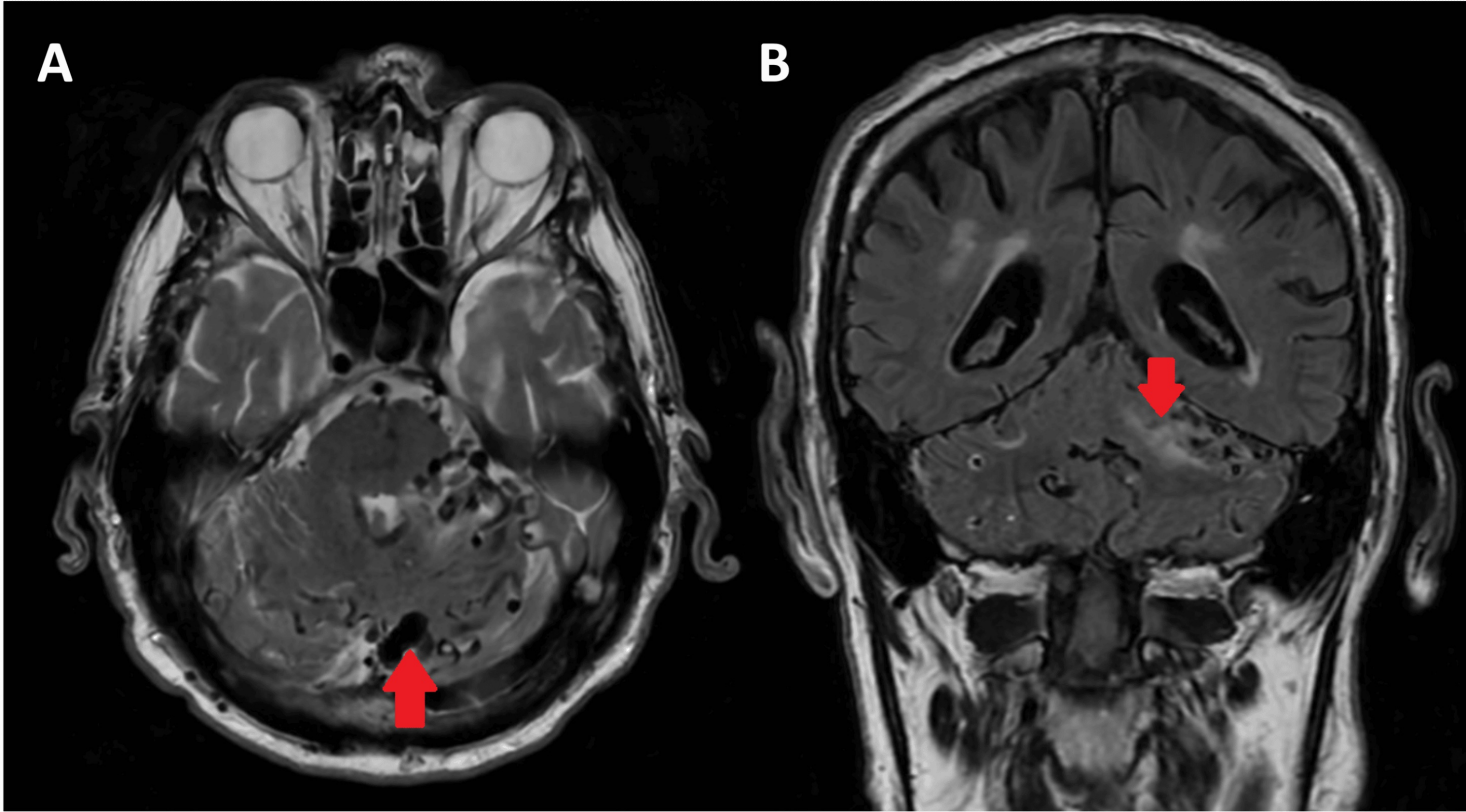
Initial MRI of the patient’s head. (A) Axial T2-weighted imaging demonstrates numerous dilated veins in the cerebellar hemispheres, vermis (red arrow), and pons. (B) Coronal fluid-attenuated inversion recovery (FLAIR) imaging demonstrates high-signal oedema (red arrow) in the left cerebellar hemisphere.
